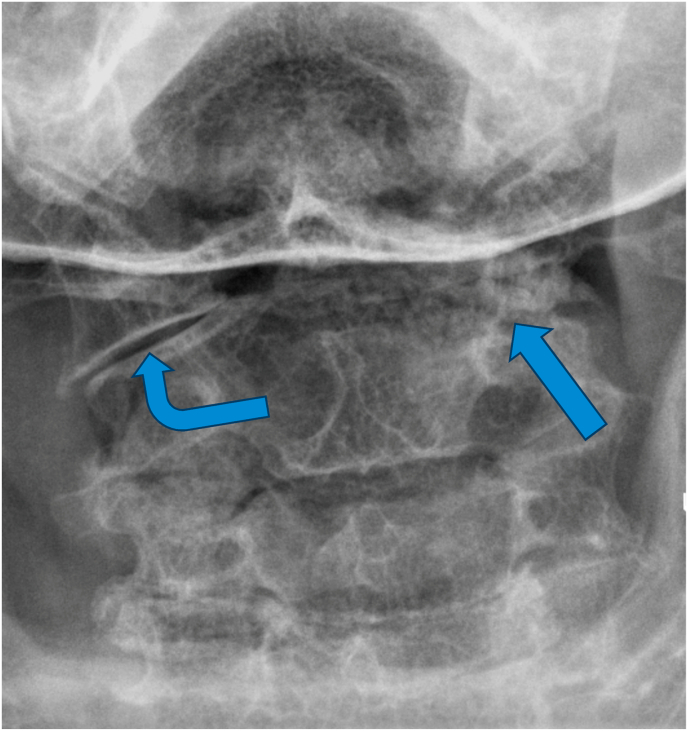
X-ray from a patient with left suboccipital pain demonstrating severe left C1-2 joint arthrosis (straight arrow) with a well-preserved joint on the right side (curved arrow).
